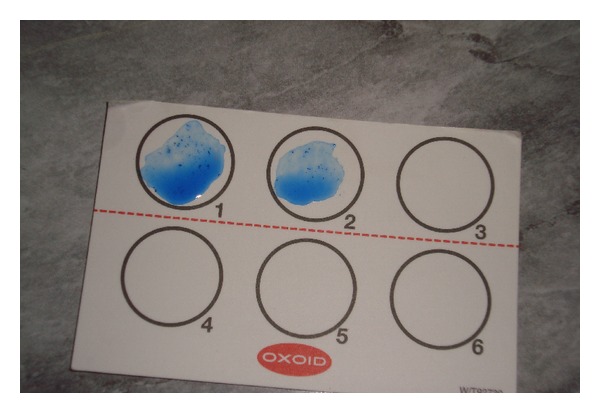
Agglutination of *E. coli *O157 and H7 latex reagent with isolates colonies of nonsorbitol-fermenting colonies on CT-SMAC medium.
